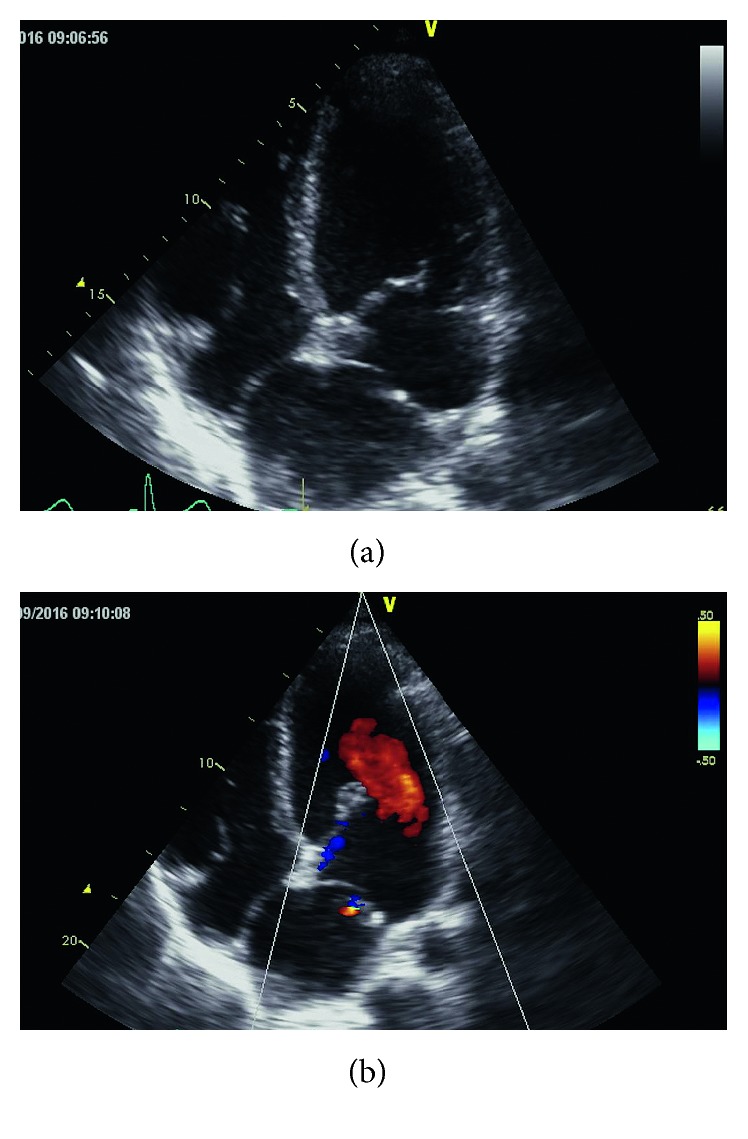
(a) An apical four-chamber view demonstrating a markedly dilated left atrium separated into two chambers by a membrane. (b) Colour Doppler images demonstrating flow across the fenestration in the membrane and across the mitral valve.
